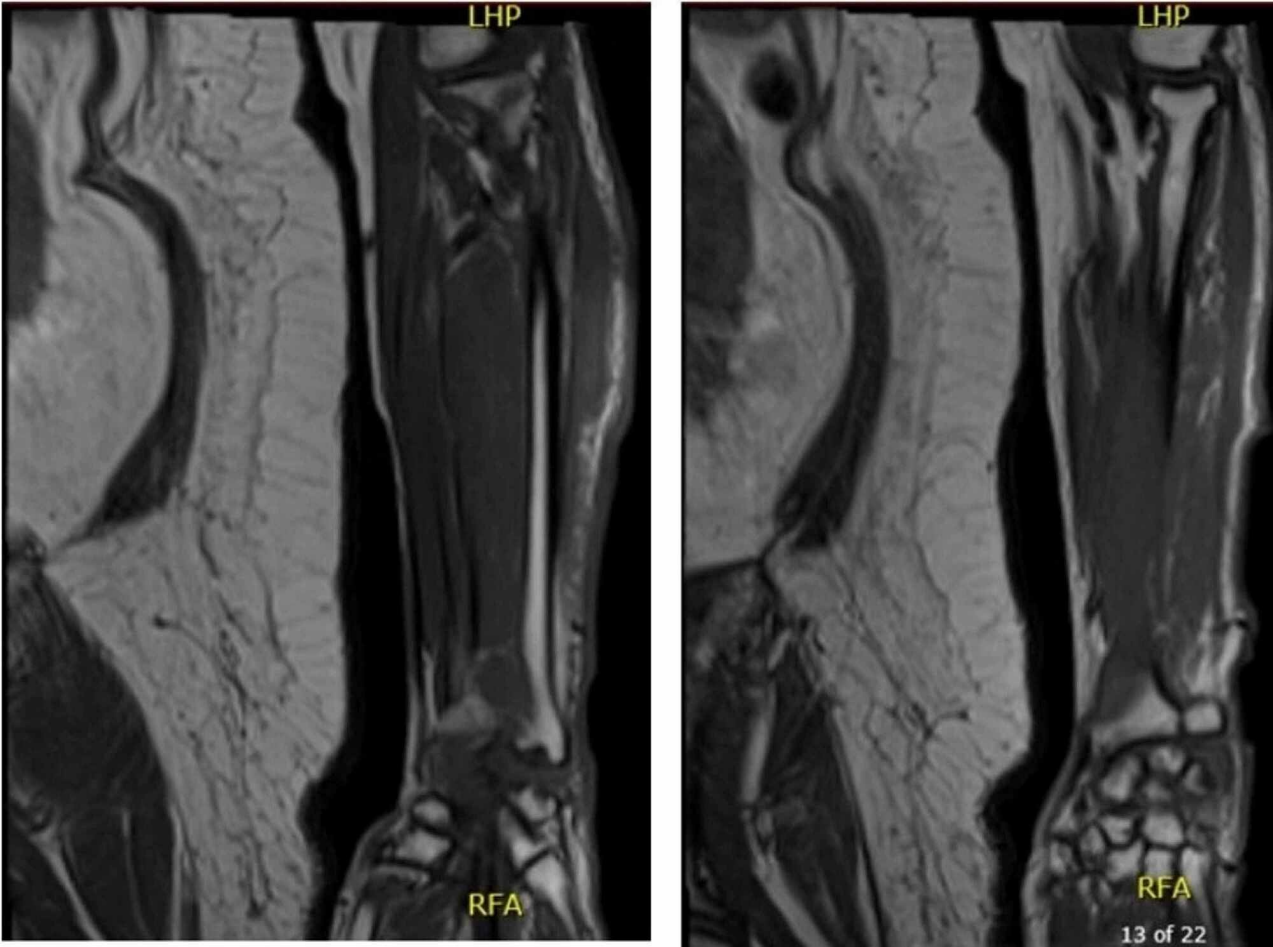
MRI with contrast of the left forearm showing cortical bone destruction and bone marrow edema with enhancing endosteum/periosteum of the radius diaphysis and metaphysis. MRI, magnetic resonance imaging
